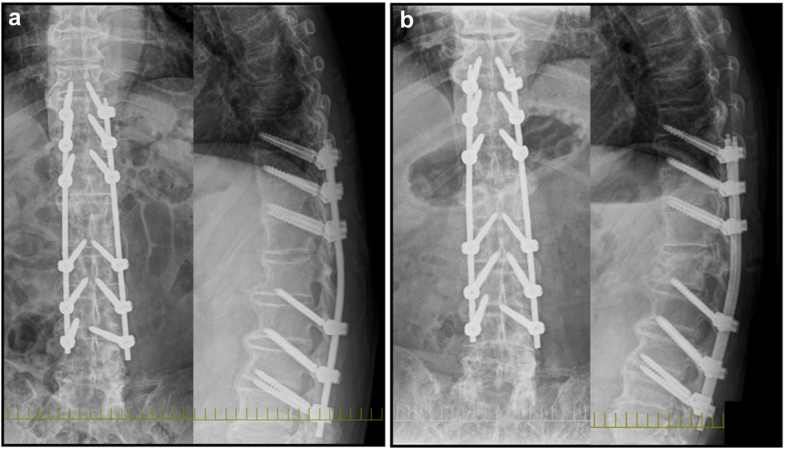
Postoperative spine radiograph after posterior spinal fusion. (a) Immediately after surgery and (b) One year after surgery.
